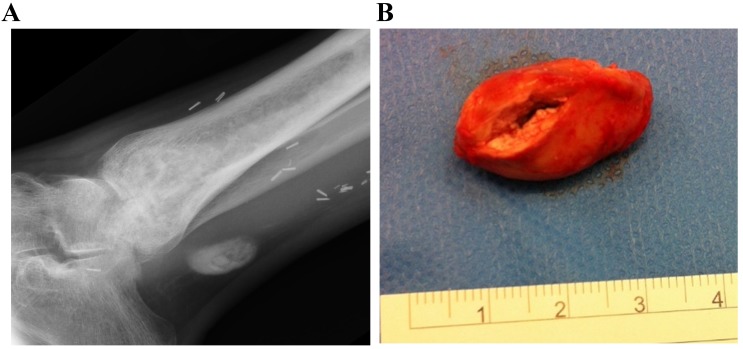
Radiograph (a) at 10 months showing a small radiodense area of extruded antibiotic carrier adjacent to the tibialis posterior tendon. The bone defect has remodelled well with no sign of recurrent infection. At operation, a 1x2x2.5cm soft mass of calcium sulphate was removed (b).
